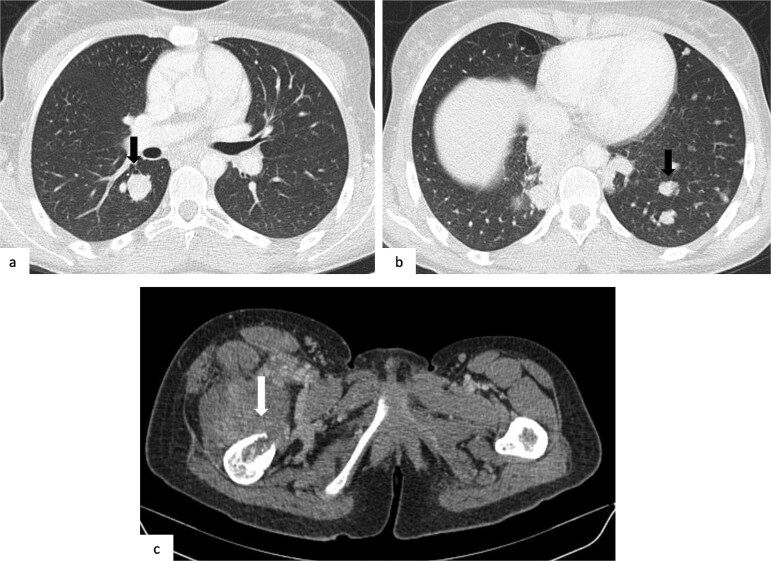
Axial contrast-enhanced CT images of the chest, abdomen, and pelvis: (a, b) thoracic CT scan in lung window showing multiple bilateral pulmonary masses and nodules, randomly distributed, dense with smooth contours (black arrows); (c) abdominopelvic CT scan revealing a lytic lesion centered on the right femoral neck, breaking through the cortex (white arrow).
